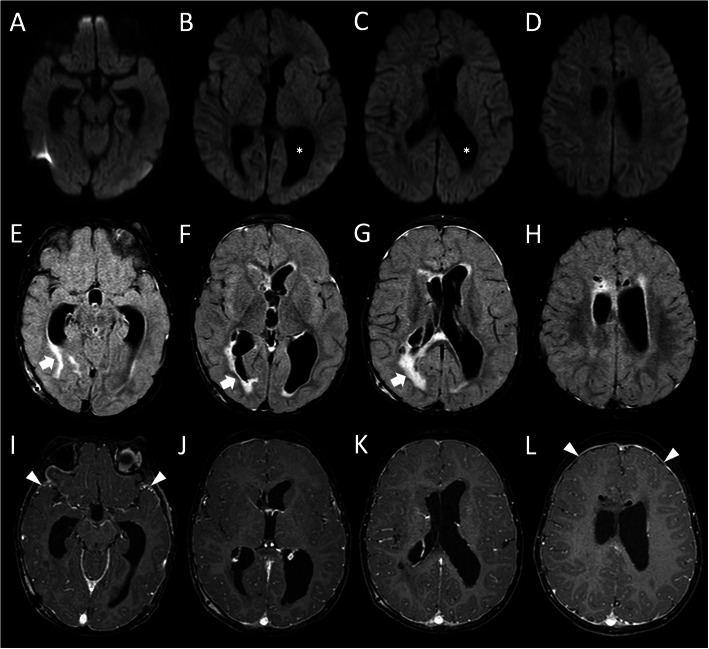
Brain MRI performed 1 month after clinical onset. a-d Axial diffusion weighted images show resolution of the signal abnormalities along the ventricular ependyma and reduction of the ventricular size after bilateral shunting procedures (asterisks). Axial contrast-enhanced FLAIR (e-h) and T1-weighted (i-j) images demonstrate marked reduction of the periventricular white matter signal alterations (thick arrows), and almost complete resolution of leptomeningeal contrast enhancement with re-expansion of the subarachnoid spaces. Note the presence of mild reactive dural contrast enhancement in the temporo-frontal regions (arrowheads)
